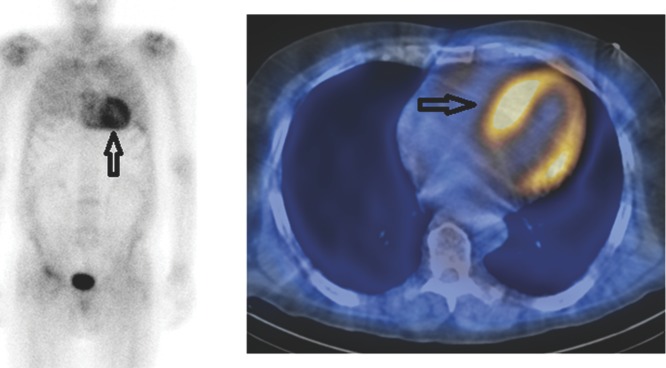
A positive 99mTc-DPD scan for TTR cardiac amyloid (left), showing uptake in the heart (arrow) and reduced bone uptake. The right-hand panel shows a fused CT/SPECT image showing myocardial uptake with greater uptake in the septum.
